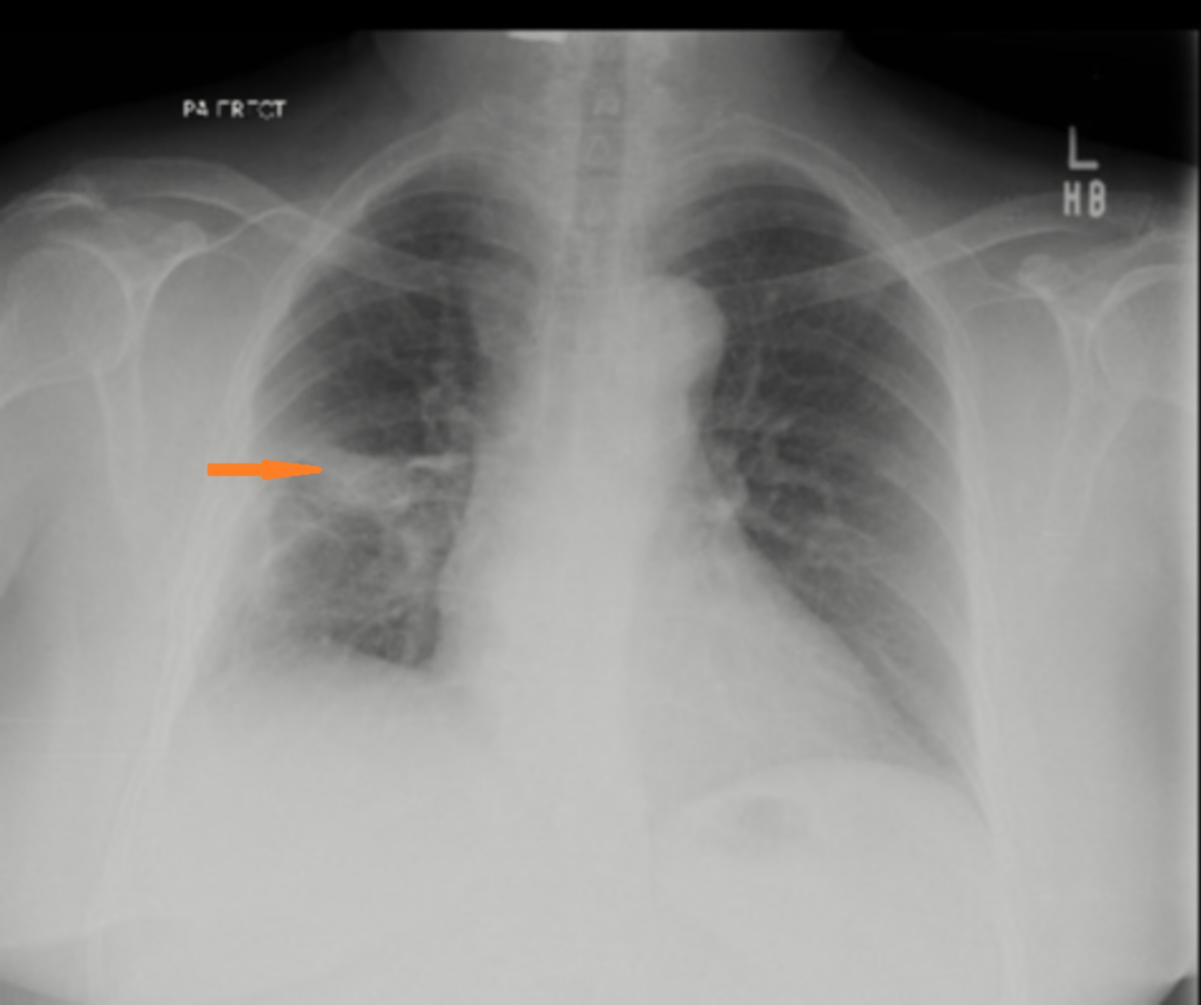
Chest X-ray after treatment showing fibrotic changes (arrow) at right middle zone.
